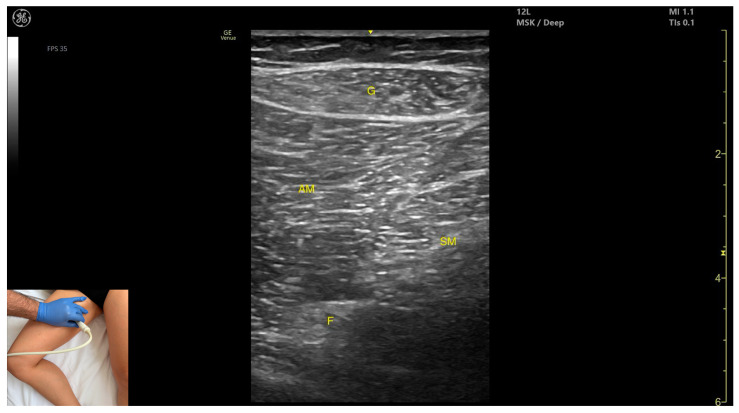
Ultrasound anatomy of *gracilis* (G) with key landmarks: G—gracilis; AM—*adductor magnus*; SM—*semimembranosus*; F—femur.
